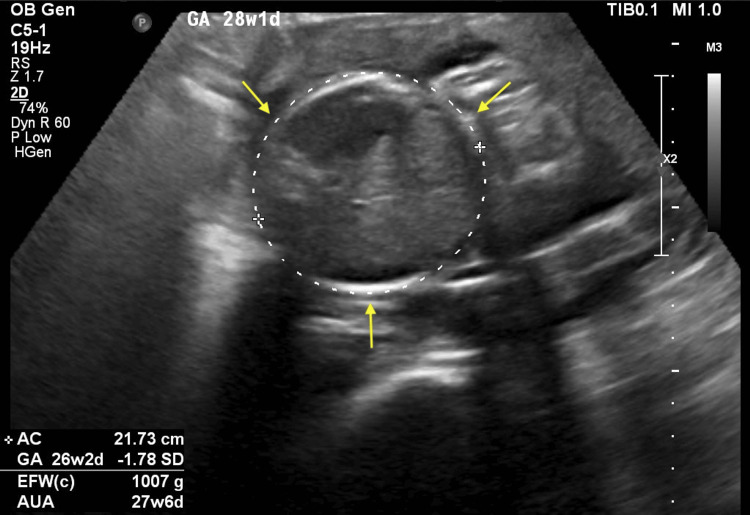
Obstetric ultrasound showing fetal abdominal circumference measuring 21.73 cm, indicated by white dotted lines and yellow arrows. The measurement corresponds to 26 weeks and two days of gestation, indicative of IUGR. AC, abdominal circumference; GA, gestational age; EFW, estimated fetal weight; AUA, average ultrasound age
